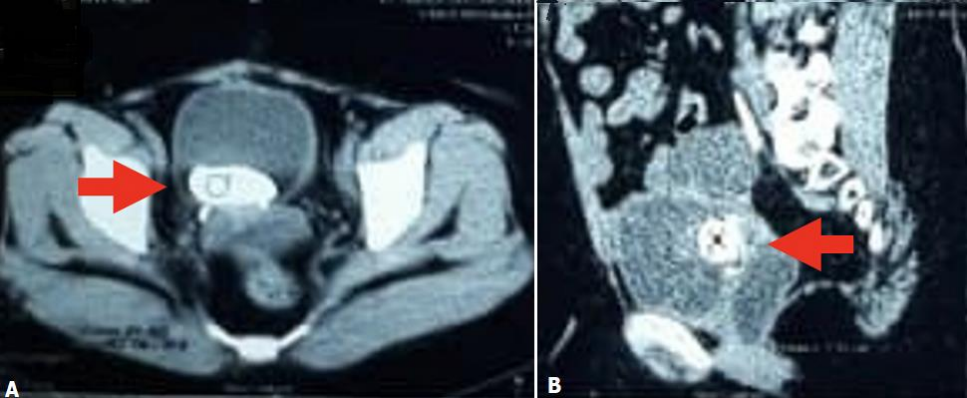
urological computed tomography scan (A, B) showed density of stone in the bladder (arrow) with size of 48 mm x 21 mm x 20 mm and the right ureteral stent in the pelvic cavity
